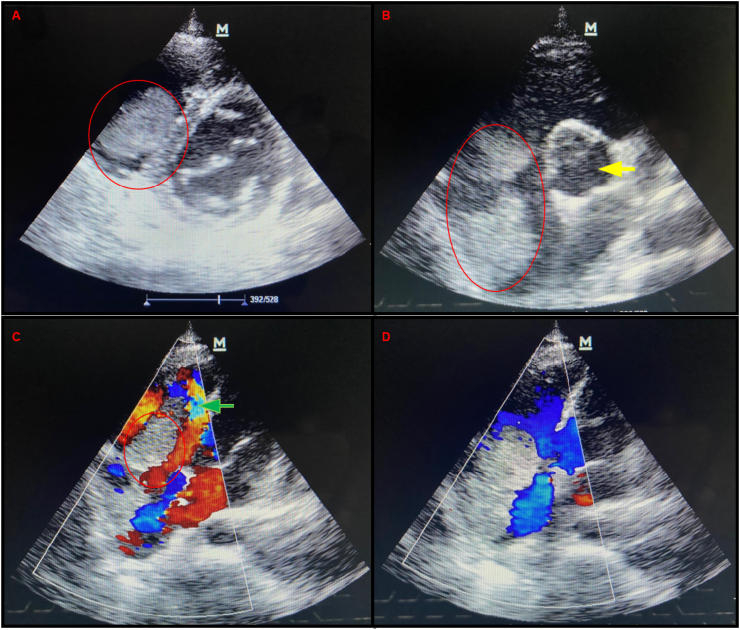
Echocardiogram. A – D shows right atrial tumour protruding through the tricuspid valve (TV) into the RV causing TV regurgitation. Red circles show tumour in the right atrium attached to the TV. Yellow arrow shows aorta in short axis. The green arrow shows mosaicism pattern due to TV incompetence. (For interpretation of the references to colour in this figure legend, the reader is referred to the Web version of this article.)
